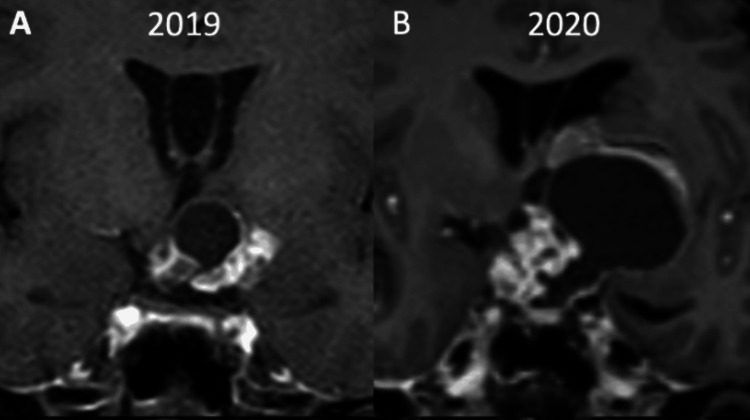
(A, B) Coronal section of post-contrast T1-weighted images obtained one year apart showing marked interval increase in the size of the lesion.
